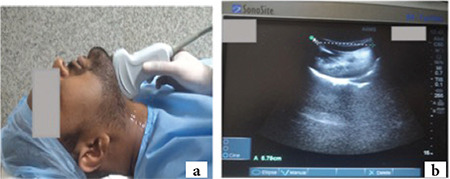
a) Second step Airway-USG-patient head in extended position with curvilinear transducer placed in suprahyoid region at midline in sagittal plane, adjusted to bring the hyoid bone and mentum in one frame. b) Ultrasonographic image at second step. A=Hyomental distance in extension. USG, ultrasonography.
